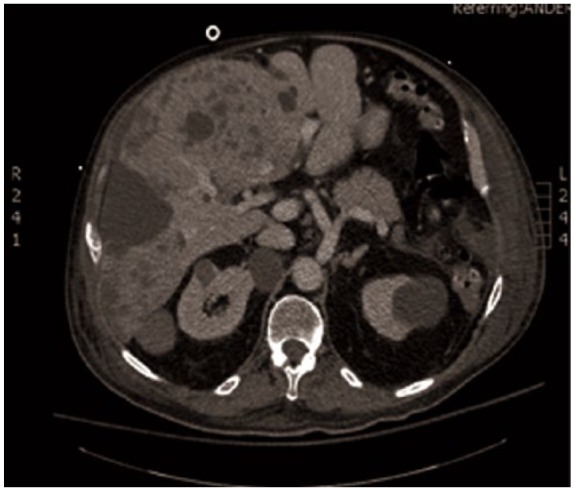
CT abdomen/pelvis with contrast obtained for evaluation of hypercortisolism. Note the presence of new low-density liver lesions not seen 3 months prior, consistent with metastases. Also, new bilateral adrenal hyperplasia.
